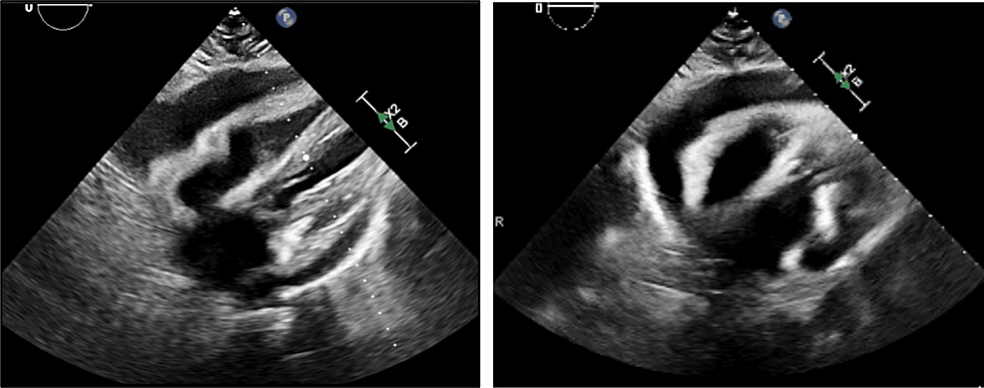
Transthoracic echocardiogram, two views, showing evidence of massive pericardial effusion along the right heart border
